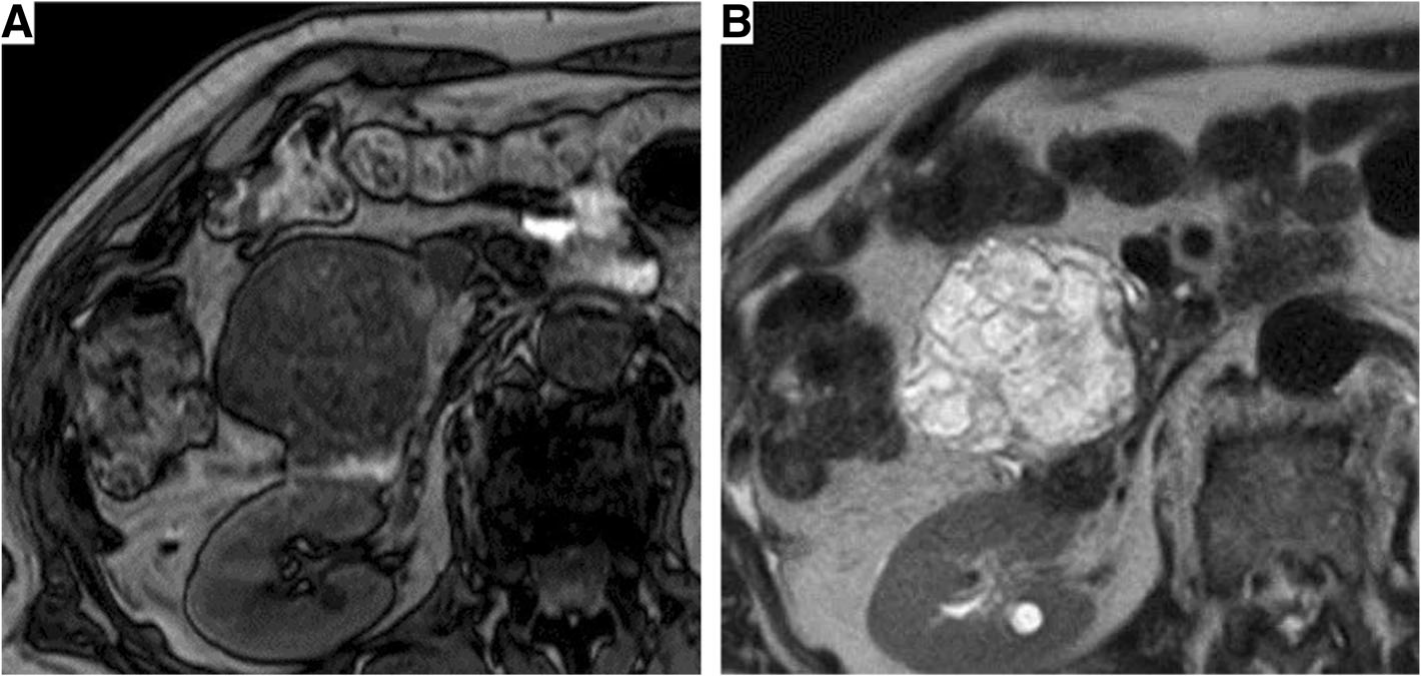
On magnetic resonance imaging, the microcysts were hypointense on T1-weighted imaging (a) and hyperintense with the septum on T2-weighted imaging (b)
